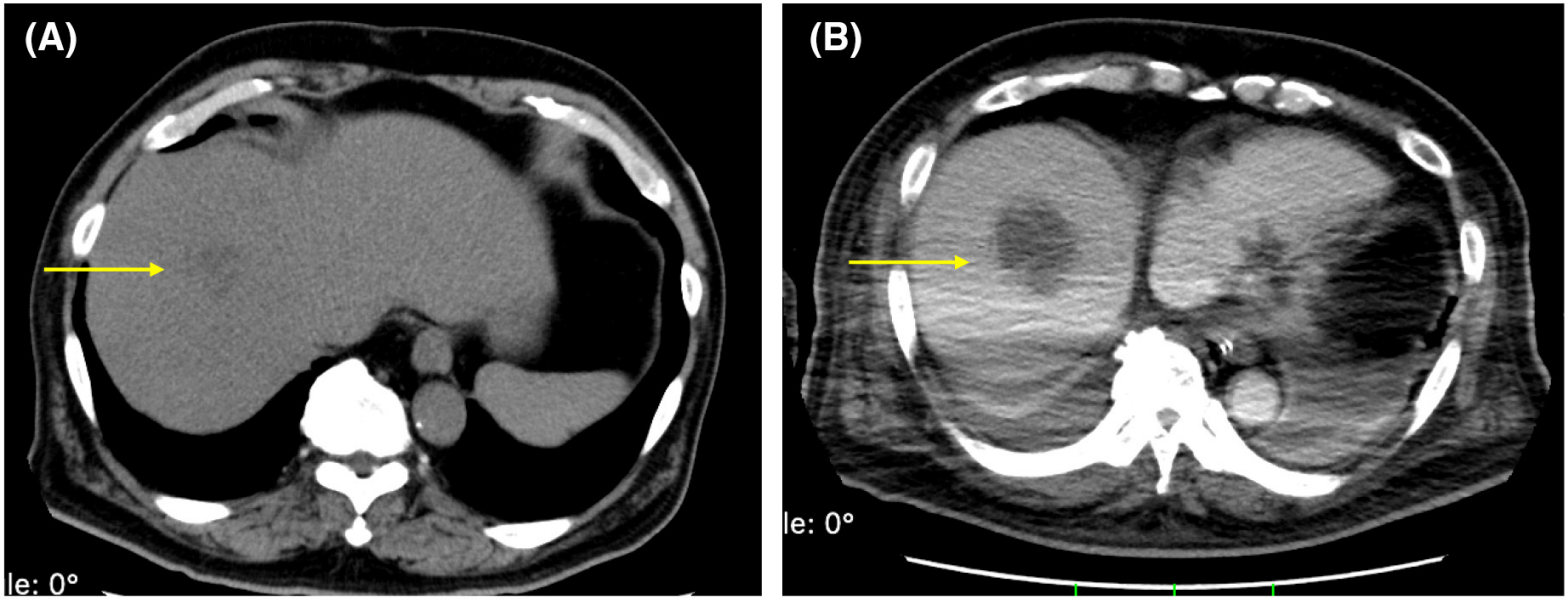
Comparison of abdominal CT scans without contrast‐enhancement on admission (A) and abdominal CT scans with contrast‐enhancement the 10th hospital day (B). The liver abscess grew from 26 (A) to 47 mm (B).
